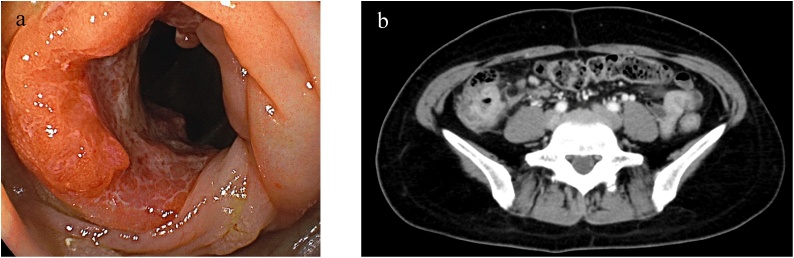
Images of the colon tumor. (a) Total colonoscopy reveals a type 2 tumor in the ascending colon. (b) Computed tomography (CT) shows an enhanced mass in the ascending colon without distant metastasis.
